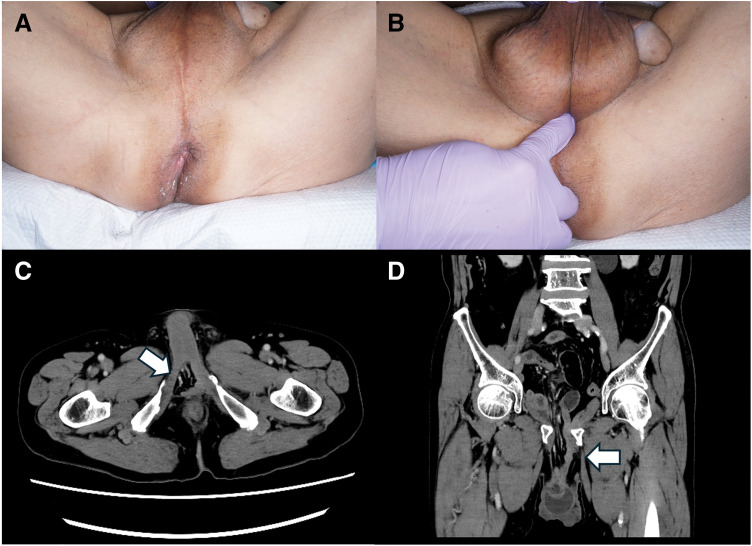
Clinical and radiological findings of the perineal hernia. (A) Supine position. No perineal protrusion is observed as the hernia is spontaneously reduced. (B) Digital palpation. A finger is easily admitted into the hernia orifice, confirming a distinct pelvic floor defect. (C, D) CT. Axial (C) and coronal (D) scans reveal the small bowel herniating anterior to the rectum and sliding into the scrotum.
